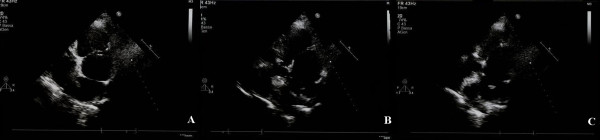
Transthoracic echocardiogram: apical 4-chamber view showing a fibromuscular membrane subdividing the left atrium into proximal and distal chambers (PANEL A). Lipomatosis of the interatrial septum, with the typical "dumbbell" aspect is evident as well (PANEL B and C).
